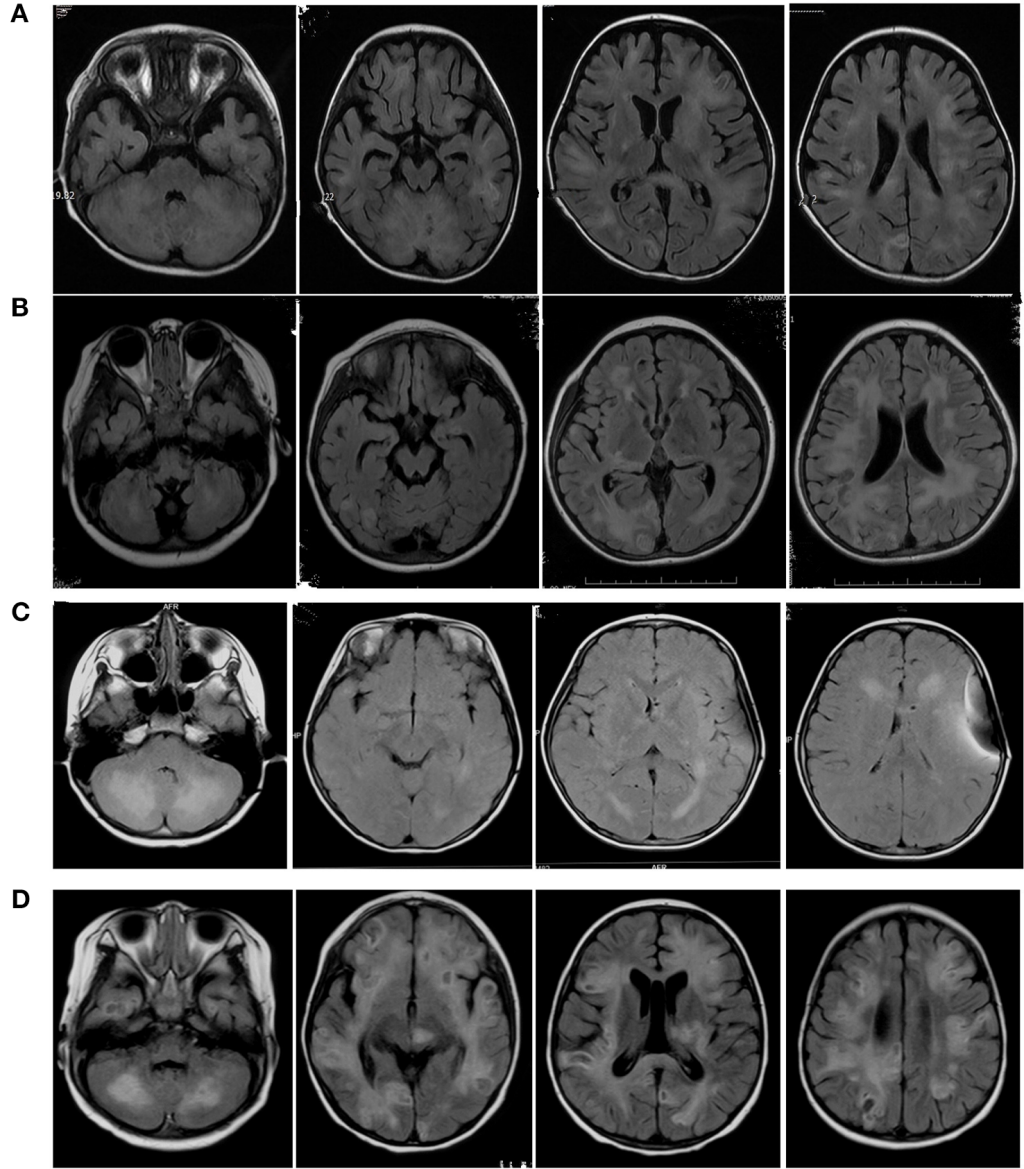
Axial Flair sequences of brain MRIs of Case1 (A), Case2 (B), Case3 (C), Case4 (D). The FLAIR-MRI revealed numerous variable size in the cerebral hemisphere, basal ganglia, cerebellum and brain stem as well as hypersignal intensities on T2-MRI.
